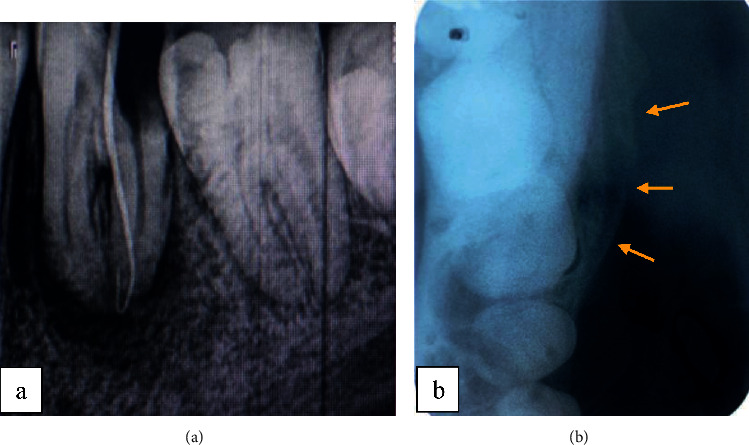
(a) Periapical radiograph revealed a not limited periapical lesion. (b) Occlusal radiograph revealed a periostitis reaction in relation with the external cortex.
